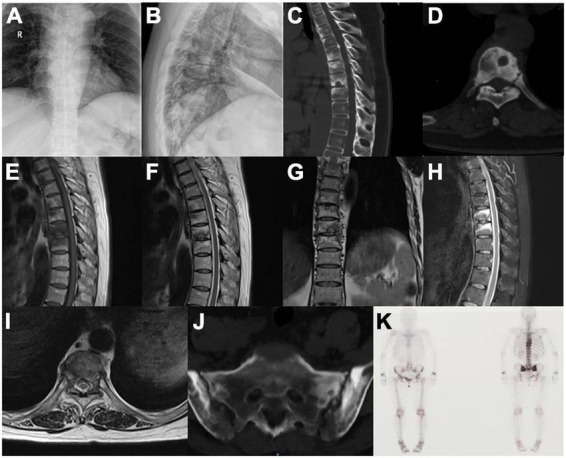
Osteoarticular manifestations of SAPHO syndrome (A–K). (A,B) Plain radiographs revealed heterogeneous density of thoracic vertebrae and osteophytes at the edges of thoracic vertebrae. (C,D) Thoracic CT scanning demonstrated cortical erosion, osteophyte, and osteosclerosis of thoracic vertebrae. (E–I) Thoracic MRI showed thoracic vertebrae with vertebral lesions. (J) Sacroiliac joint CT revealed unsmooth joint surface, extensive sclerosis of the bone and patchy low-density shadow. (K) Whole-body bone scintigraphy demonstrated multifocal osteoarthritic lesions.
